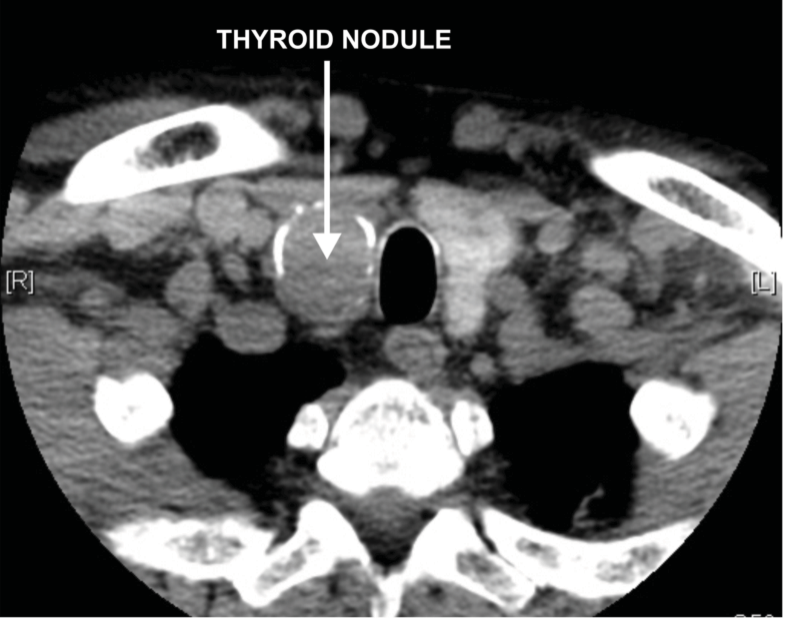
CT scan demonstrating 4.3 × 2.5 cm partially calcified complex dominant nodule in the inferior pole of the right lobe of thyroid.
